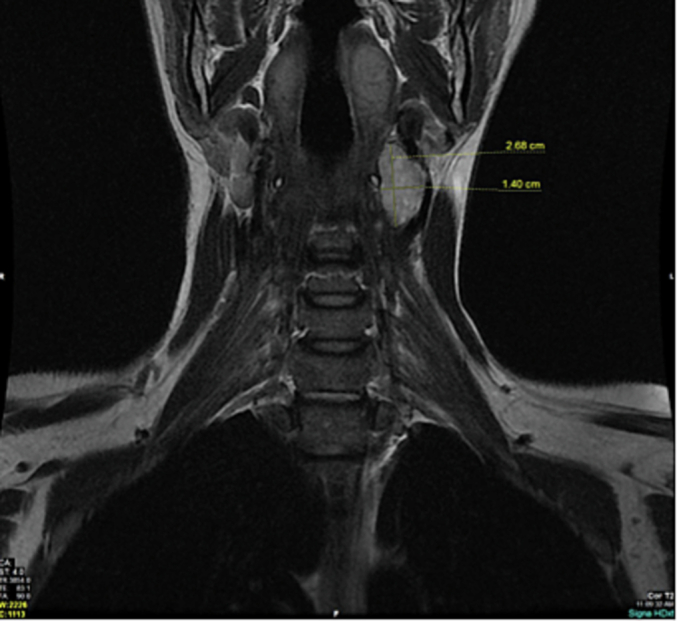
Coronal image of MRI showing well defined oval lesion at the carotid bed, showing intermediate to high signal on T2 fat spin echo sequence. Abutting the surrounding vasculature with mild mass effect. Measuring (1.4 × 1.4 × 2.68 cm –on AP, ML, CC dimensions).
